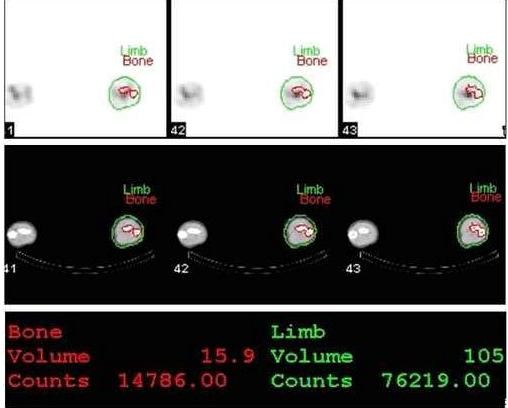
SPECT/CT reconstruction with X-ray image showing volume (in ml) and counts in the bone (red) and total limb (green).
